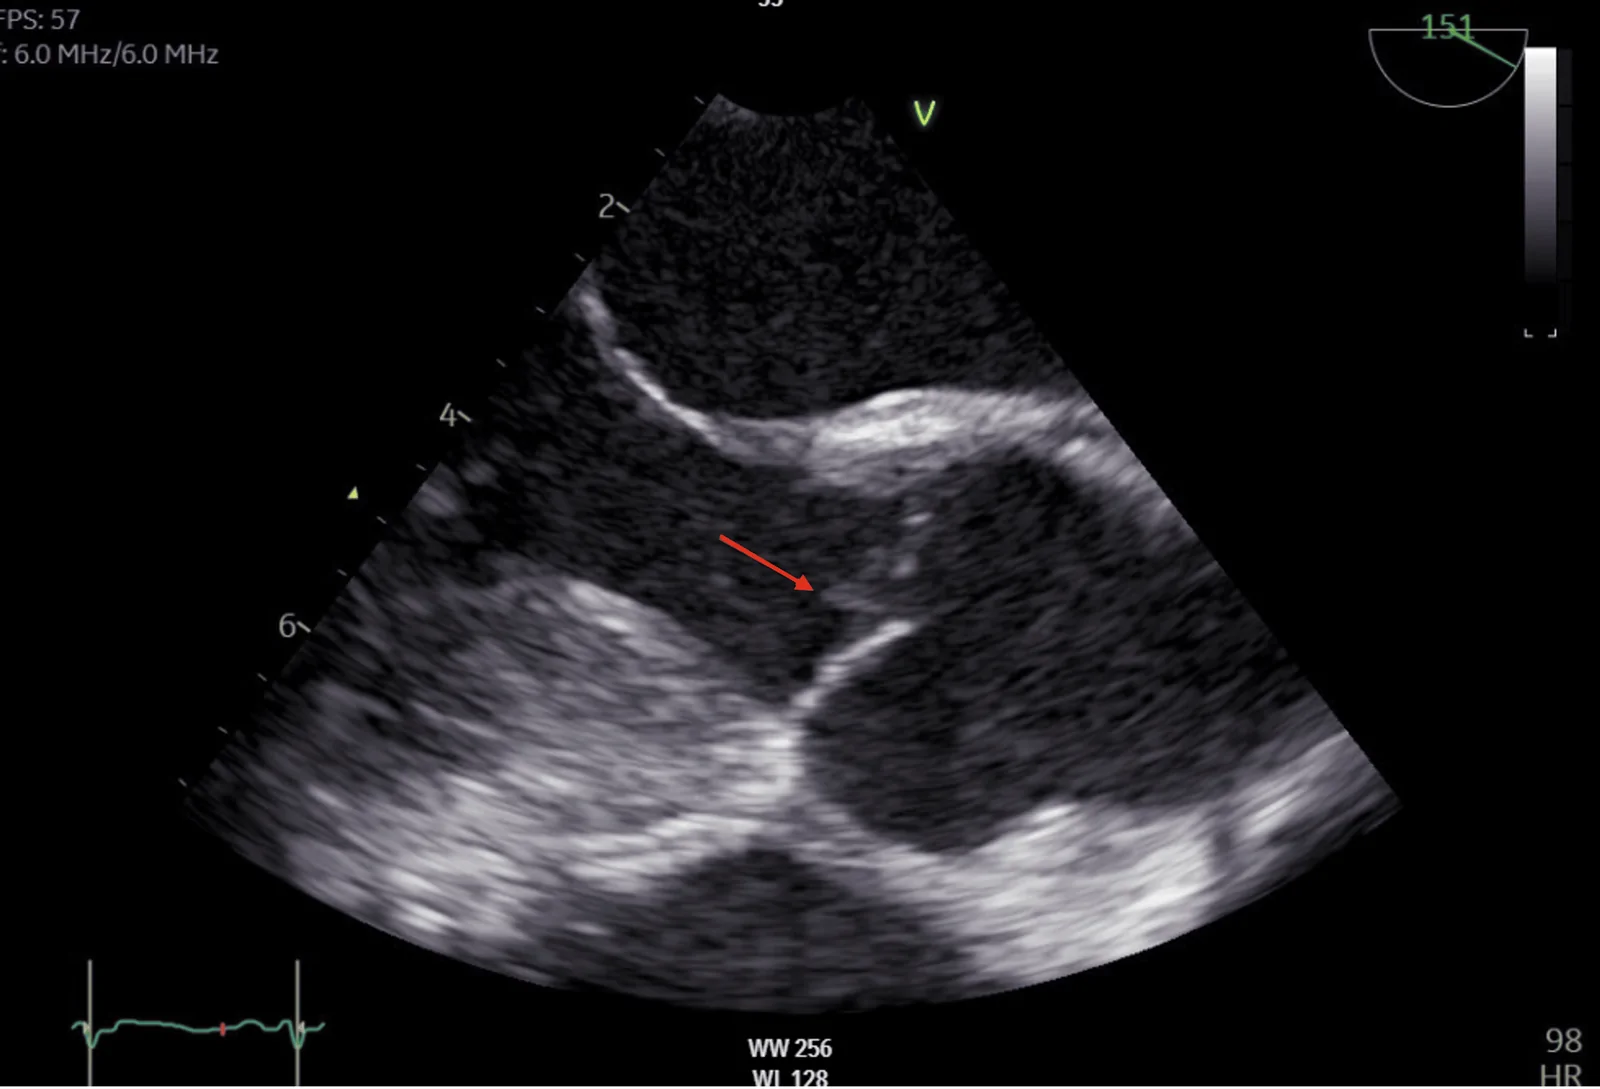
Transoesophageal echocardiography (TOE) findings: still image from the TOE demonstrating a 9 × 7 mm echogenic structure attached to the right coronary cusp of the aortic valve The red arrow points to the 9 × 7 mm echogenic structure attached to the aortic valve, which appears grey on the image.
